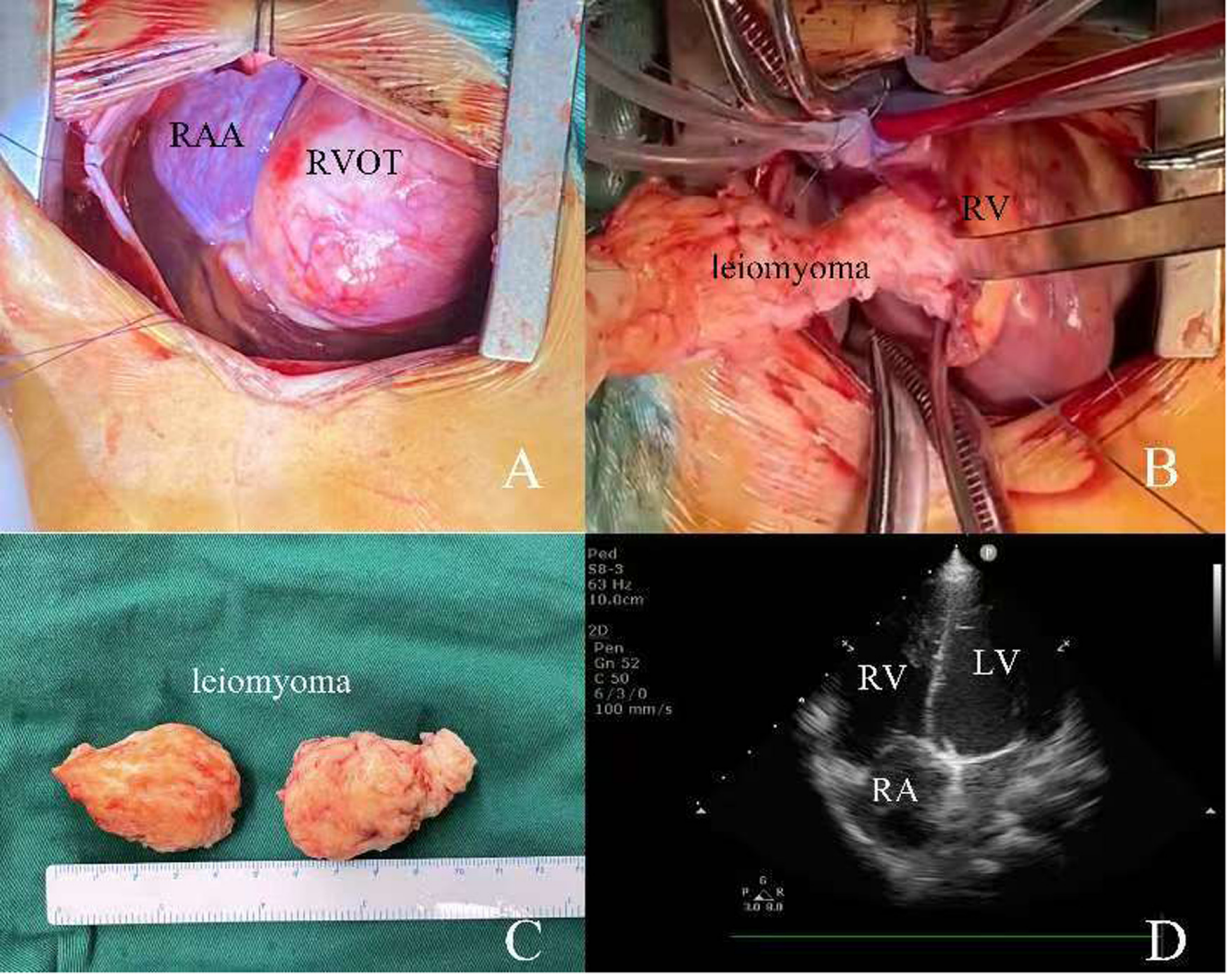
(A,B) intraoperative view of RVOT and the resection of leiomyoma; (C) the size of resected leiomyoma; (D) transthoracic echocardiography after surgery. RAA, right atrial appendage; RA, right atrium; RV, right ventricle; RVOT, right ventricular outflow tract.
